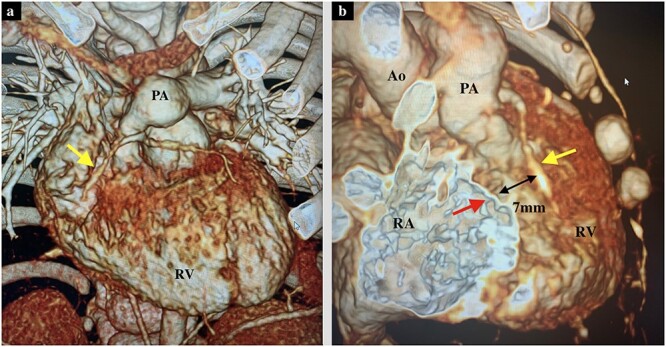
(a) Preoperative 3D-computed tomography of the axial view showing PS and the RCA (yellow arrow). (b) Preoperative 3D-computed tomography of the right oblique view showing the distance between tricuspid valve annulus (red arrow) and RCA (yellow arrow) of 7 mm. Ao, Aorta; RA, right atrium.
